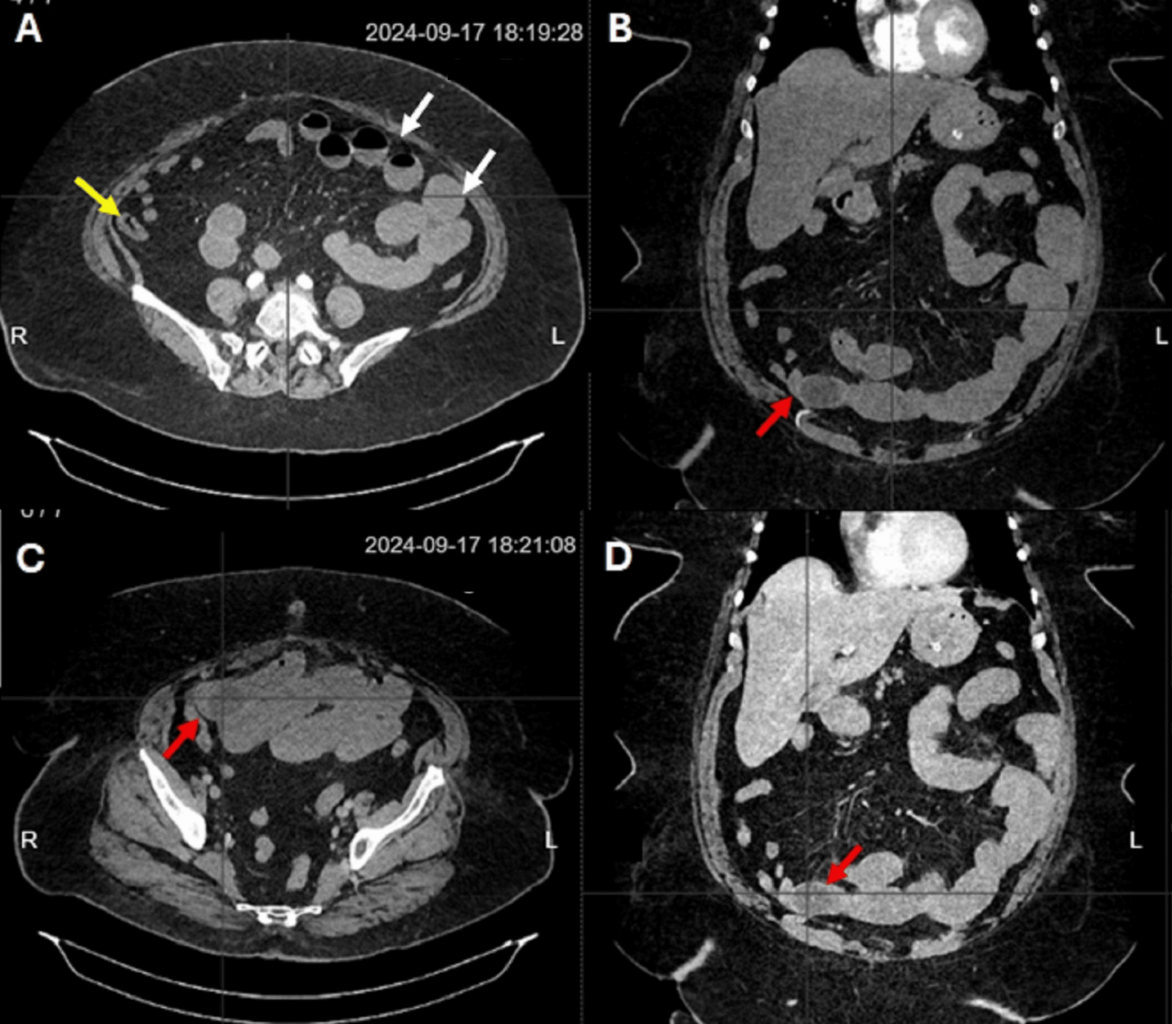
Contrast-enhanced abdominal CT showing signs compatible with distal intestinal obstruction. (A) Dilated small bowel loops with air-fluid levels (white arrow). The colon appears collapsed (yellow arrow), suggesting small bowel obstruction. (B, C, and D) Presence of a foreign body in the distal ileum causing a transitional zone (red arrow).
